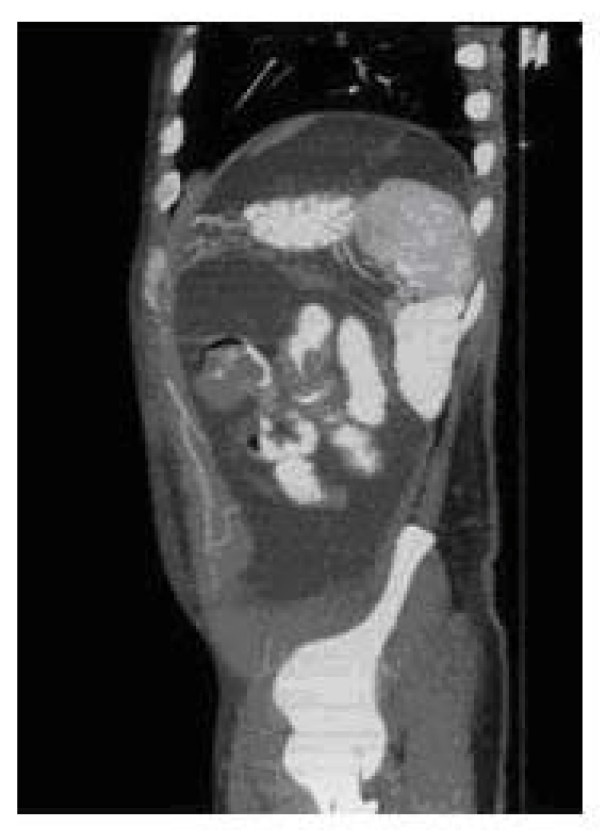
Abdominal CT scan reveals multiple nodular masses in mesentery and small bowel causing luminal narrowing (sagittal section).
